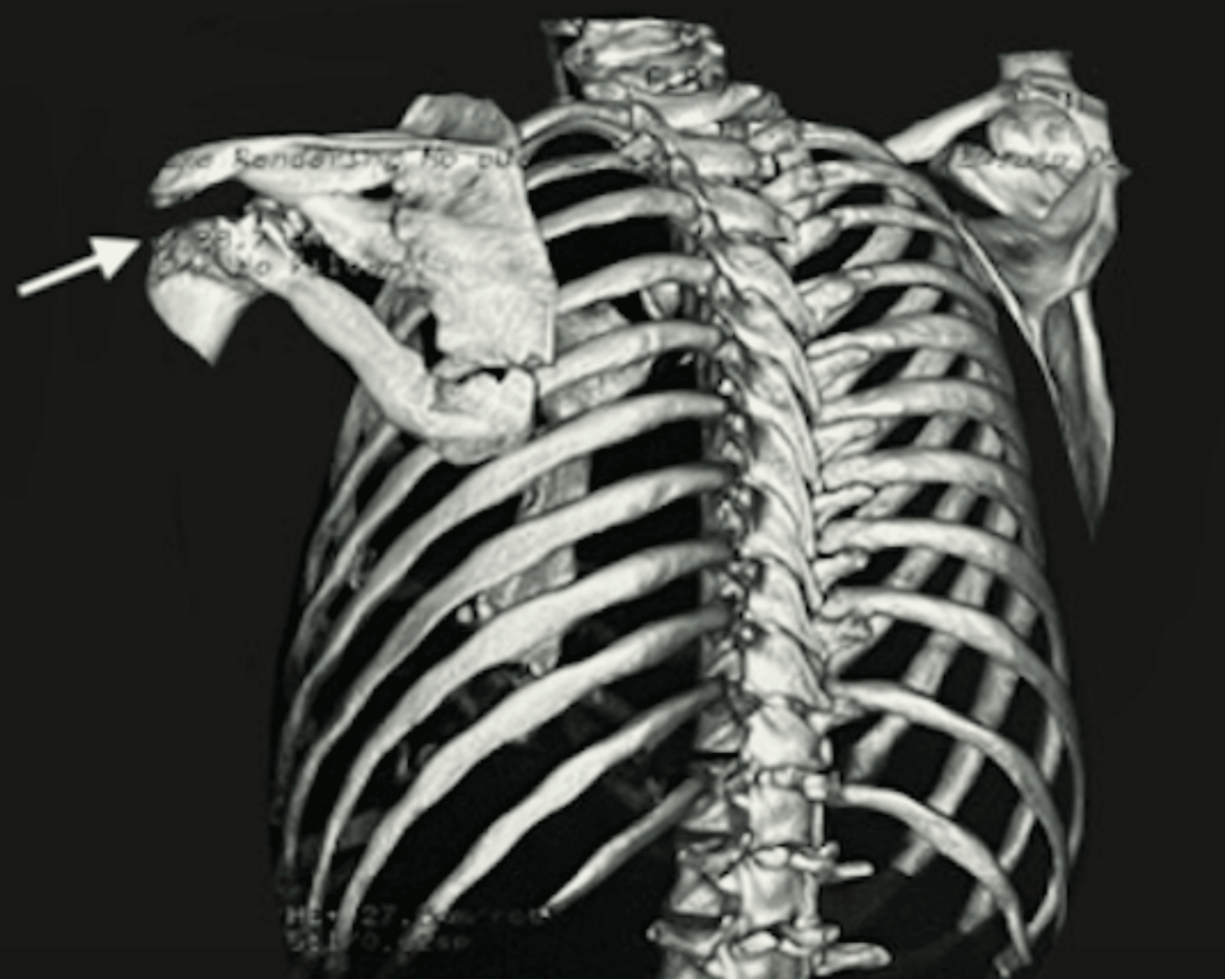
Thoracic CT bone reconstruction with evidence of multifragmentary fracture involving both the scapular body and the glenoid cavity. CT: computed tomography
